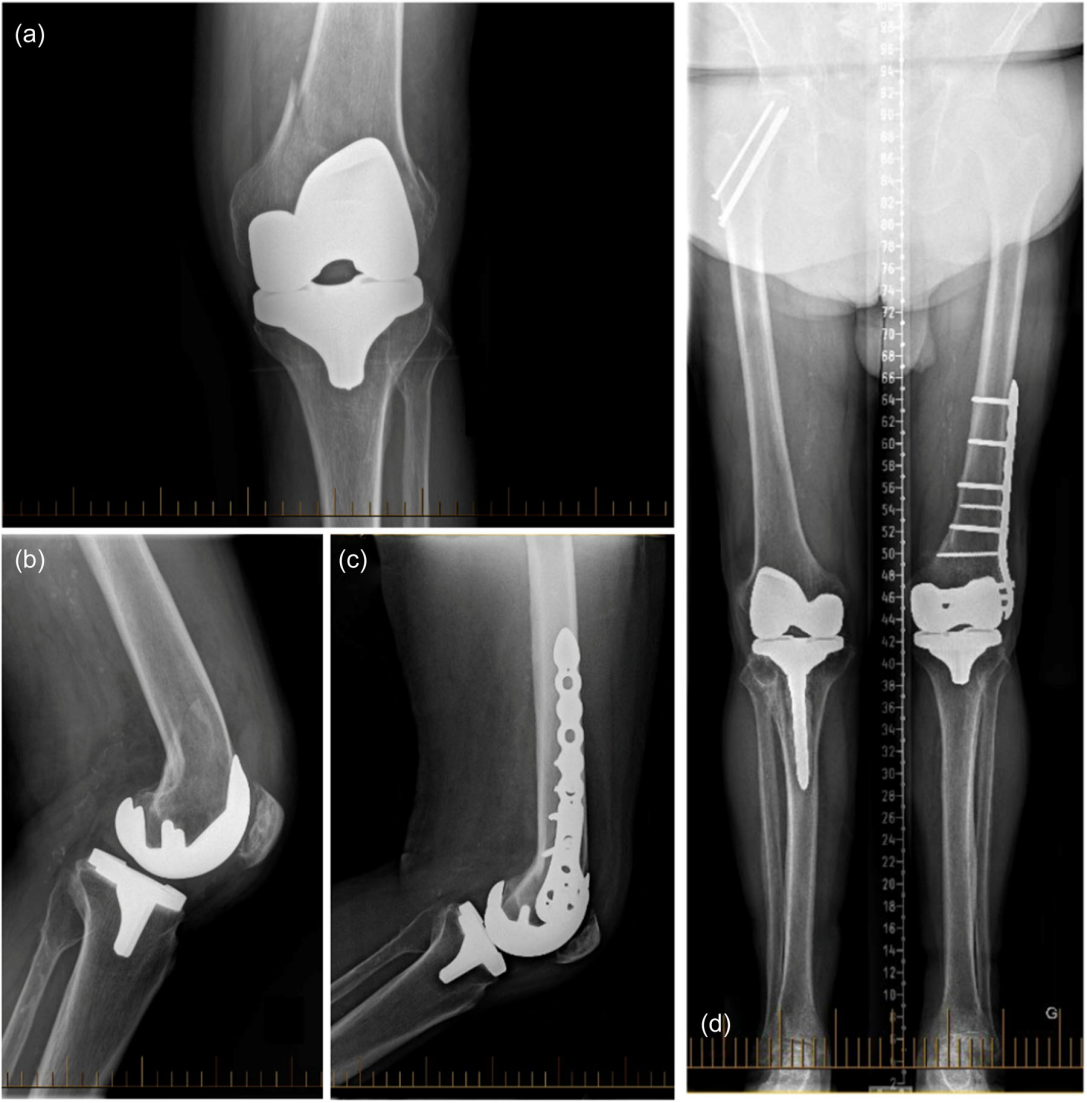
Periprosthetic TKA fracture. Anteroposterior (a) and lateral (b) radiographs of a periprosthetic femur fracture. Anteroposterior (c) and lateral (d) radiographs after open reduction and internal fixation with a lateral distal femur plate without implant replacement. TKA, total knee arthroplasty.
